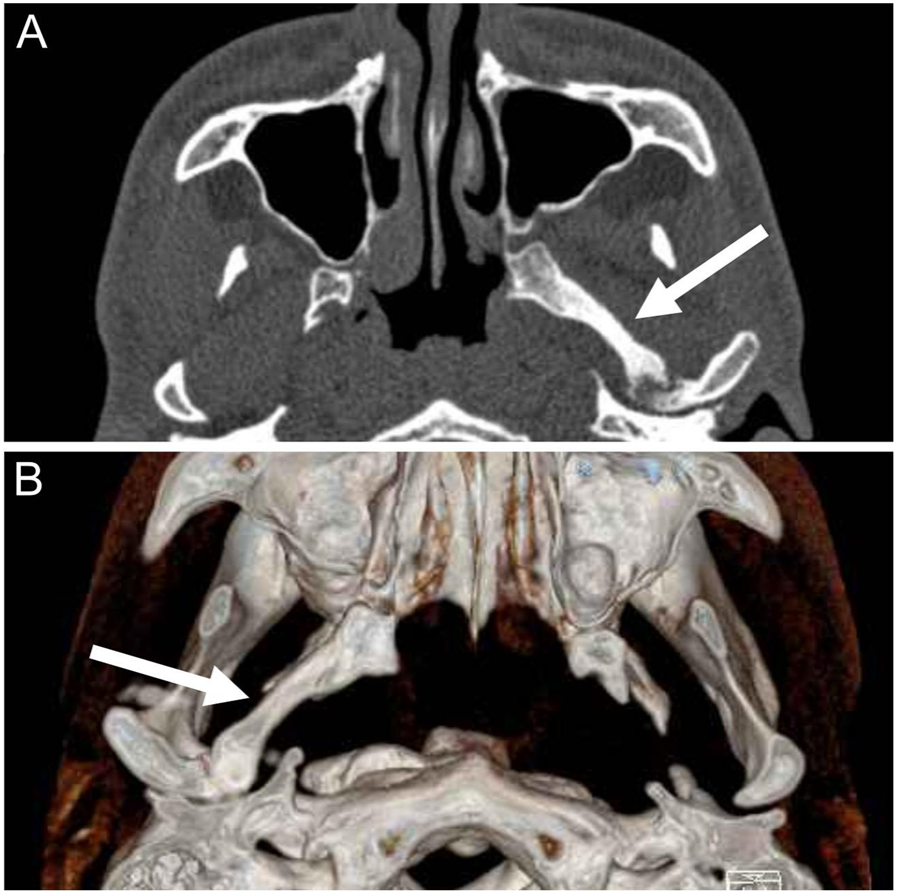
Post-physiotherapy (12 months) computed tomography scan. (A) Axial projection, upper arrow pointing the calcifications of the left lateral pterygoid muscle; (B) three-dimensional volume rendering, lower arrow pointing the calcifications of the left lateral pterygoid muscle (upper view).
